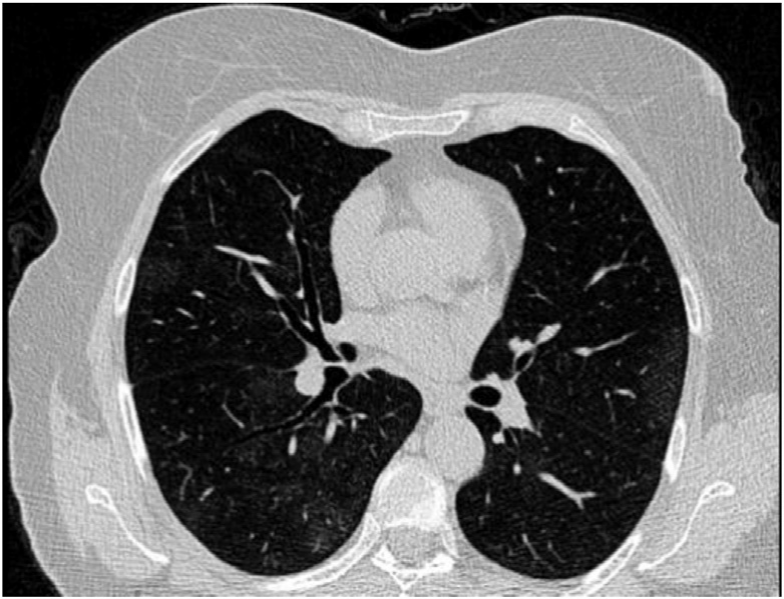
Chest CT slice of PCR positive patient (B1) with multilobar images of ground glass opacity (GGO) mainly located in the inferior lobes.
